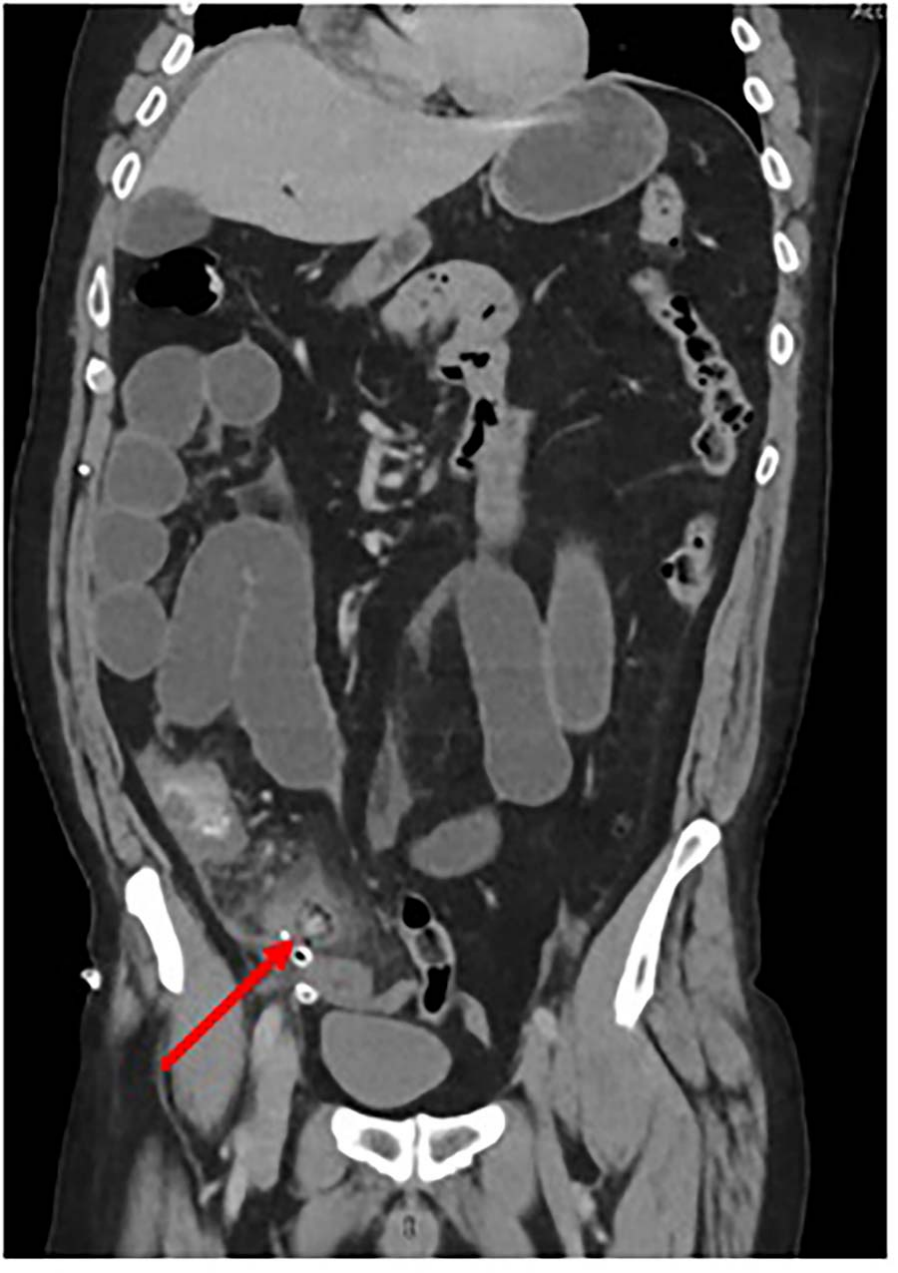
Coronal CT scan depicting Surgicel® as a tubular structure containing gas. (Created by authors)
